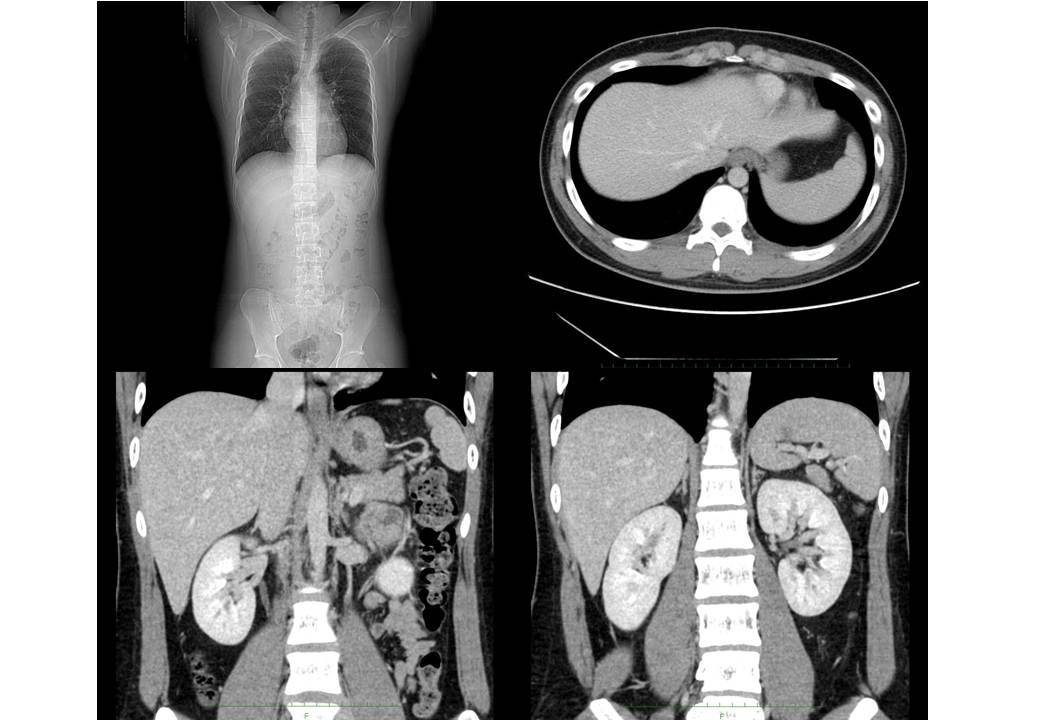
Postoperative enhanced chest and abdominal computed tomography (CT) scans. Two months postoperatively, an outpatient chest CT was performed. There was no recurrence of diaphragmatic hernia
